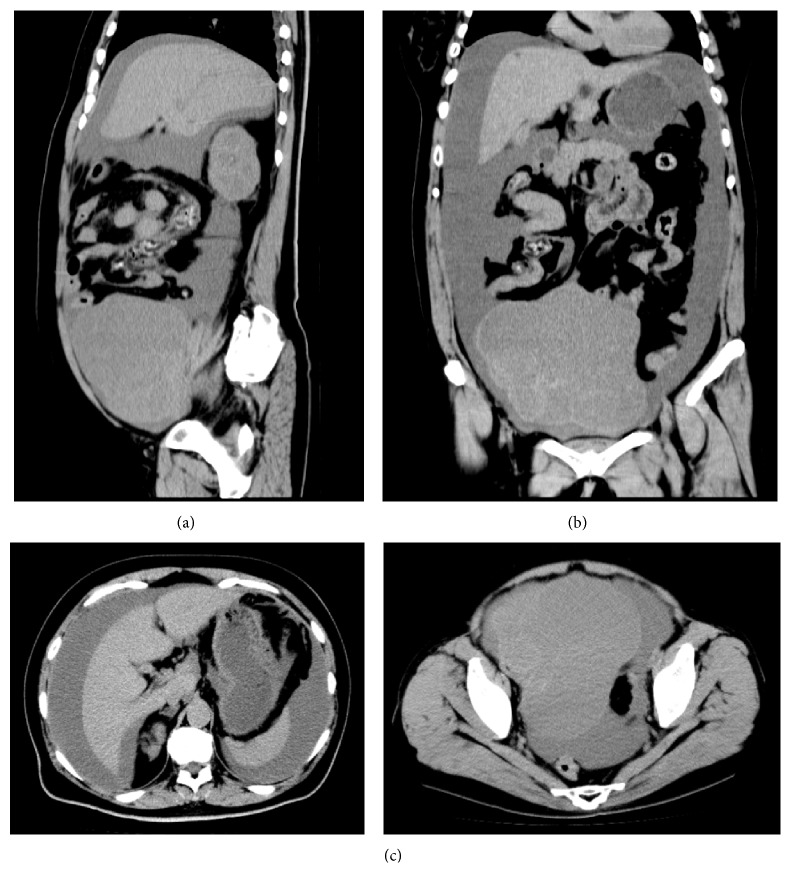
Abdominal plane computed tomography reveals a large pelvic mass with massive ascites that reach the liver and spleen. (a) Sagittal section; (b) frontal section; (c) coronal section.
